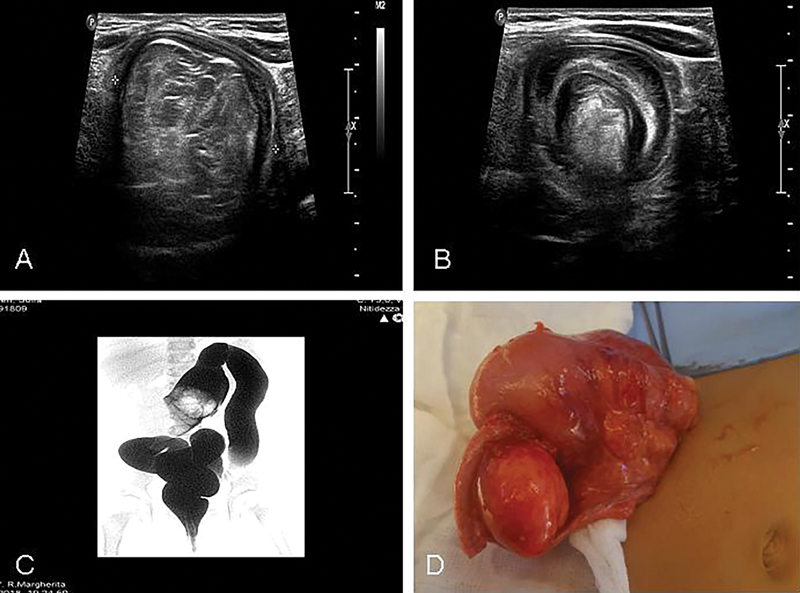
Abdominal ultrasound confirming the intussusception (
